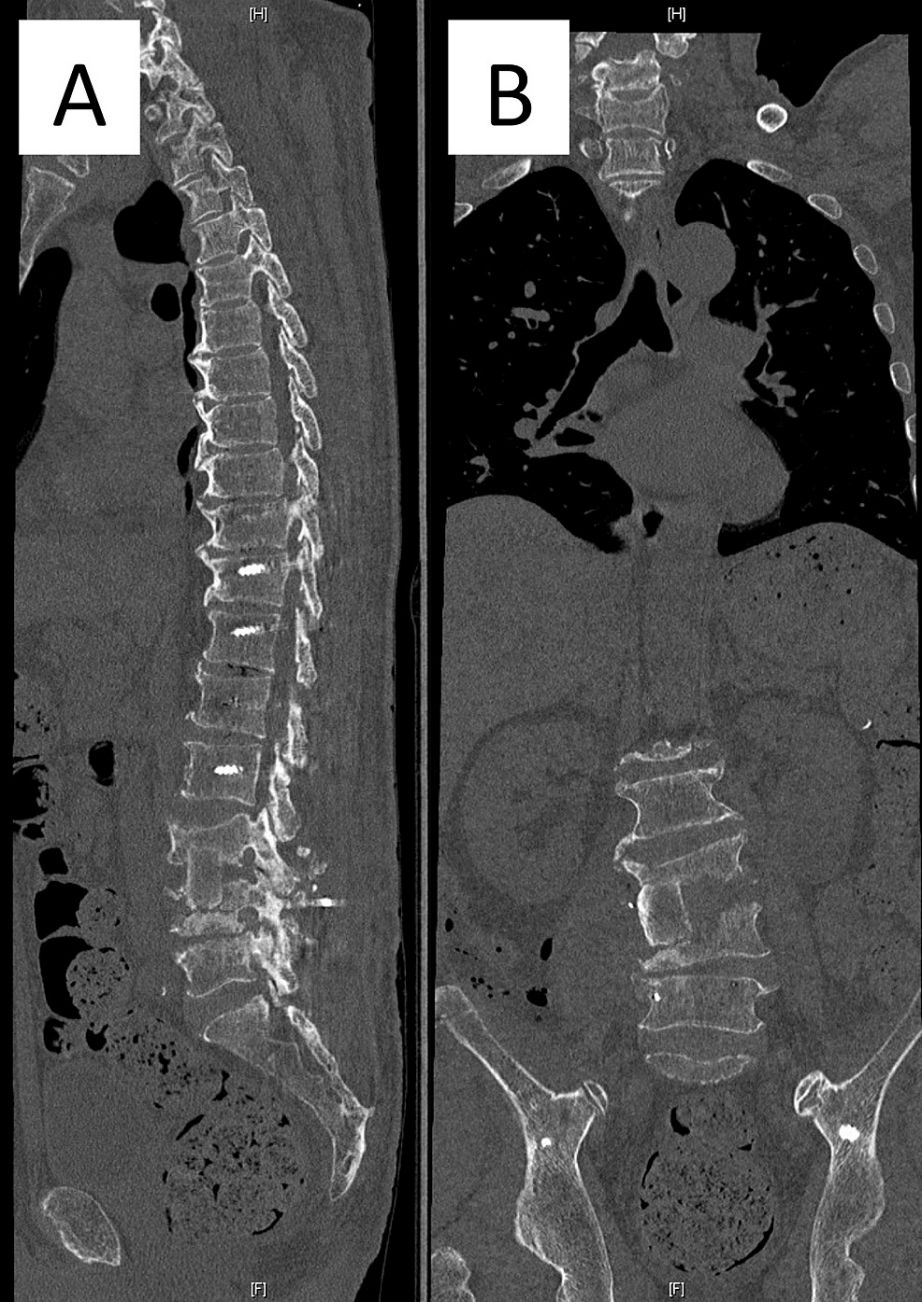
Postoperative computed tomography (CT) images of anterior debridement and autogenous bone grafting to L3/4. (A) Sagittal CT image. (B) Coronal CT image.
